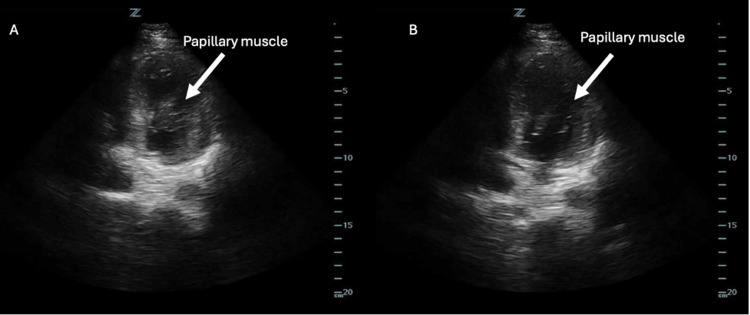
(A) An apical four-chamber view of the left ventricle obtained using a phased-array probe, which shows a papillary muscle that appears free-floating and may be misinterpreted as a thrombus; (B) The same apical four-chamber view, now revealing that the papillary muscle is connected to the ventricular wall. Image Credit: Original images by authors
